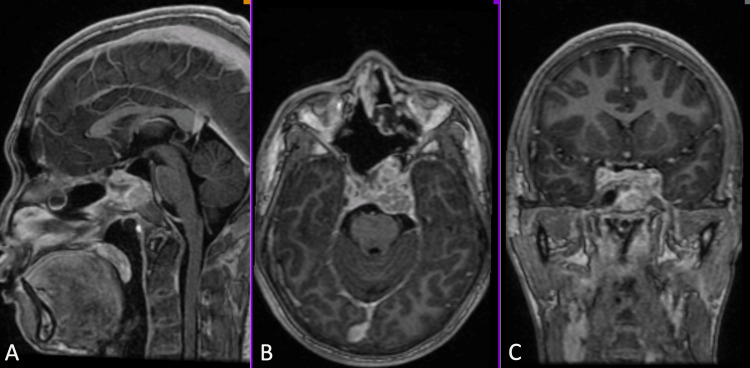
Postoperative brain MRI T1 with contrast showed a heterogeneously enhancing, lobulated mass in the clivus measuring 3.8 x 4.1 x 2.7 cm (AP x W x CC). (A) Sagittal view, (B) Axial view, and (C) Coronal view.
